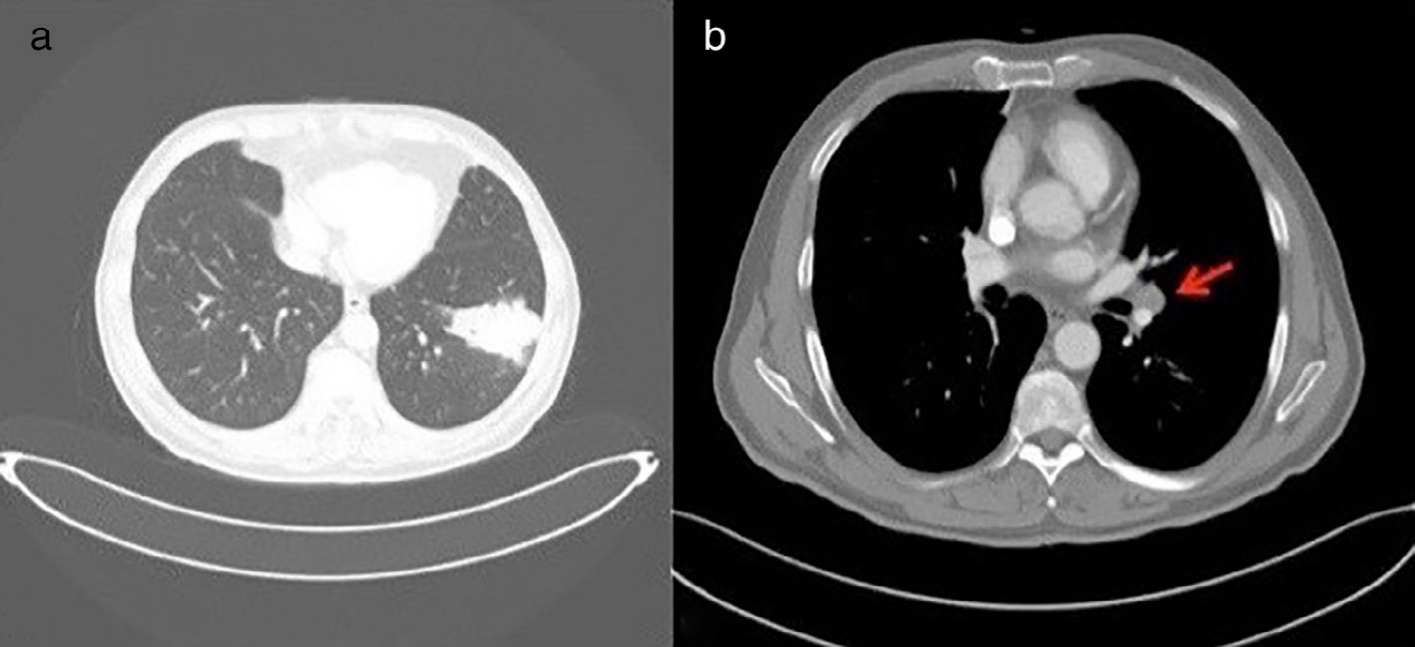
Chest computed tomography (CT) images. (a) Malignant lesion in the left lower lobe. (b) Mediastinal window revealing an enlarged interlobar lymph node (red arrow).
